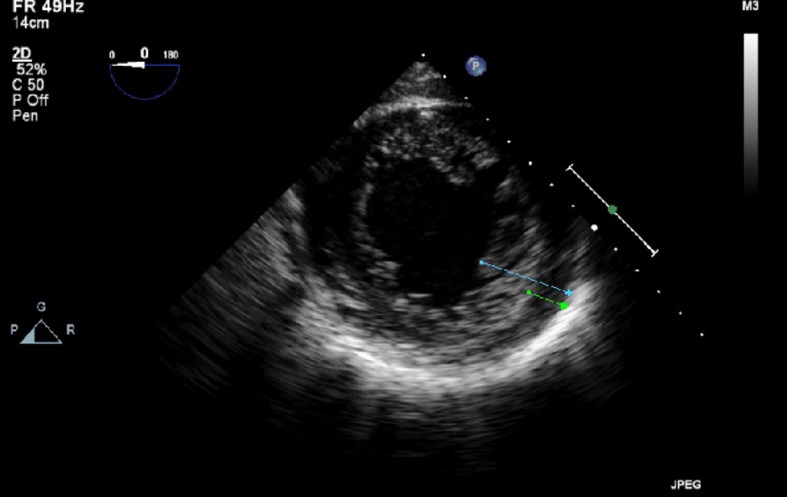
Transesophageal echocardiogram with trans gastric view without contrast shows prominent trabecular projections with the distance from the epicardial surface to the trough of the trabecular recess X (green line) of 0.85 cm, and with the distance from the epicardial surface to the peak of trabeculation Y (blue line) of 2.6 cm with ratio X/Y < 0.5.
